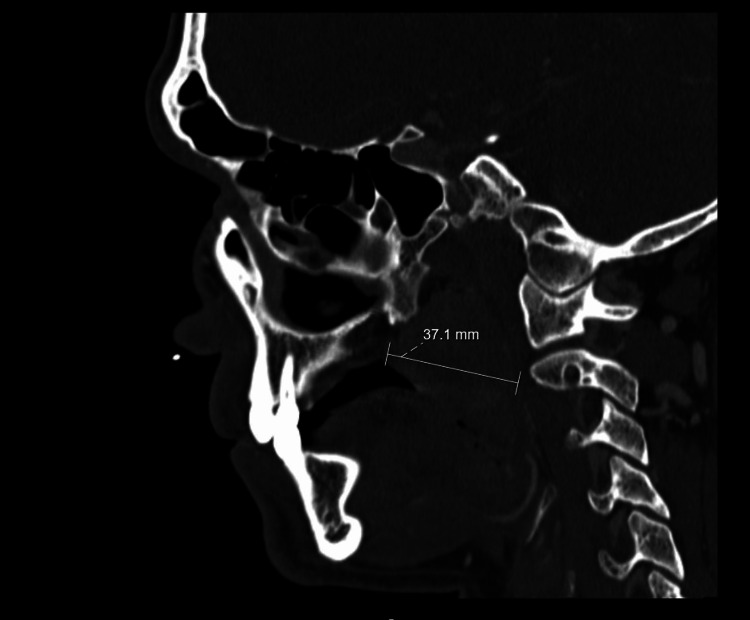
CT scan: sagittal plane (mass with approximately 37 mm). CT, computed tomography
